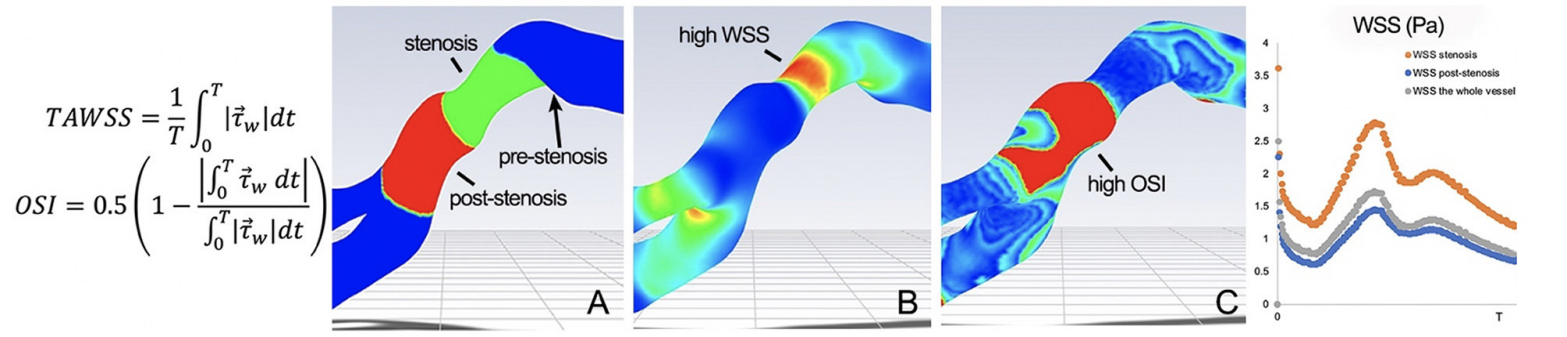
Pulsatile CTA CFD of MCA ICAD, revealing (A) a 4.9 mm stenotic segment (green) and corresponding 4.9 mm post-stenotic segment (red). The WSS map (B) depicts high TAWSS and WSSR in the stenotic segment. The OSI map (C) shows mean OSI of 0.31 in the post-stenotic segment. ${\overset{\to }{\tau }}_{w}$ represents the WSS vector and T represents the period of the cardiac cycle.
